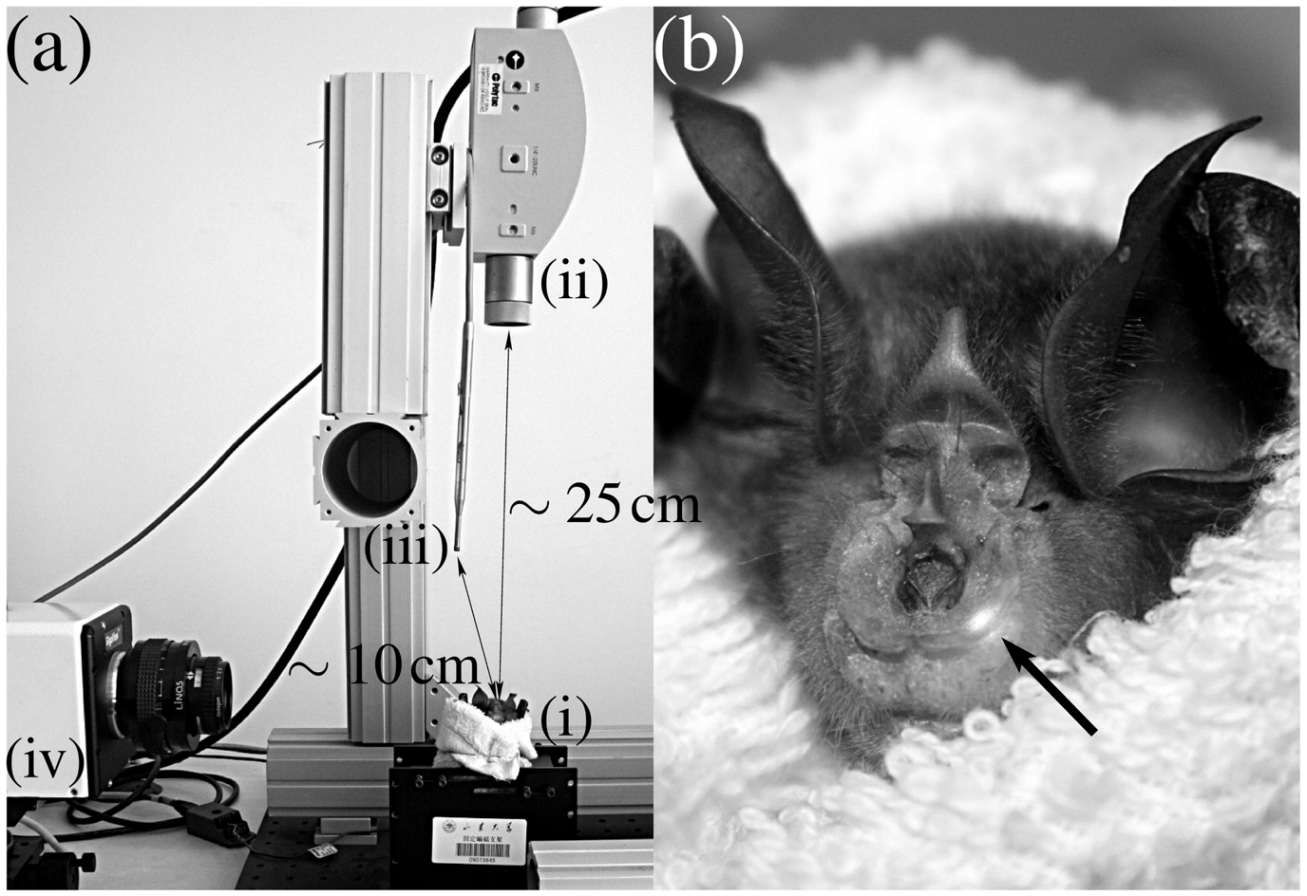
Experimental setup. a) overall layout of the setup: (i) bat, (ii) sensor head of the laser Doppler vibrometer, (iii) microphone, (iv) high-speed video camera, b) experimental subject with laser spotlight (arrow) on the anterior leaf. During experiments, the orientation of the experimental subjects was continuously adjusted by hand.
